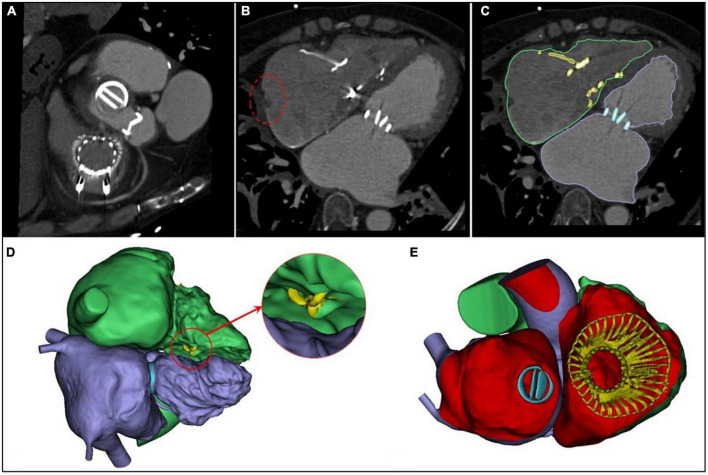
Postprocedural evaluation of the interventricular anchor. (A) A multislice computed tomography scan showed the precise positions of the two graspers. (B) The incision of the right atrium (the red circle) corresponds with Figure 1F. (C) The right heart is outlined in green; the left heart, in purple; the mechanical valve, in blue, and the LuX-Valve is yellow. (D) The yellow area in the red circle is the interventricular anchor. (E) The 3-dimensional reconstructed image from the right atrial plane demonstrates that the LuX-Valve is located in the normal position.
